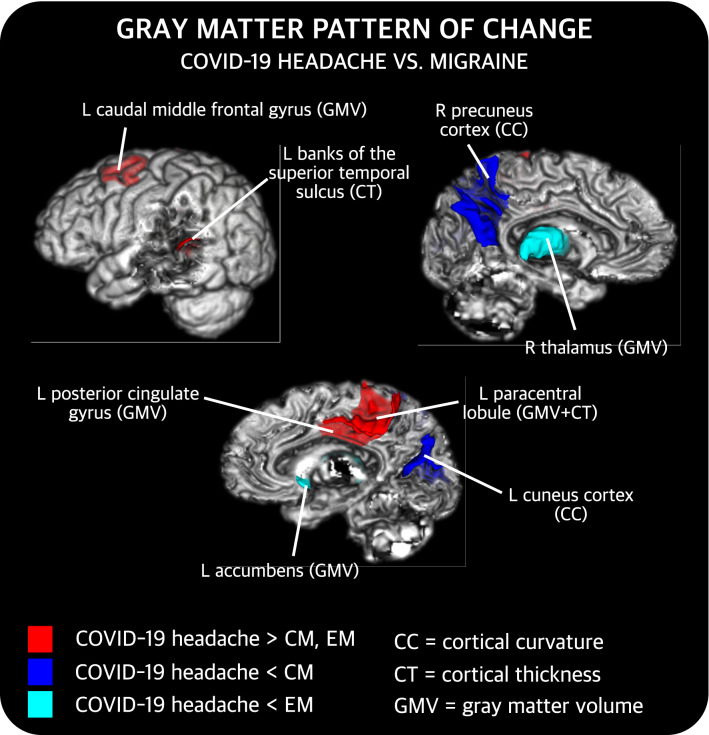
Gray matter regions that present statistically significant changes between patients with persistent headache after COVID-19 resolution (COV) and patients with episodic migraine (EM) and/or chronic migraine (CM). After false discovery rate correction, COV patients showed higher cortical gray matter volume (GMV) and cortical thickness (CT) than EM and CM, lower subcortical GMV than EM, and lower cortical curvature (CC) than CM. *L* left, *R* right
